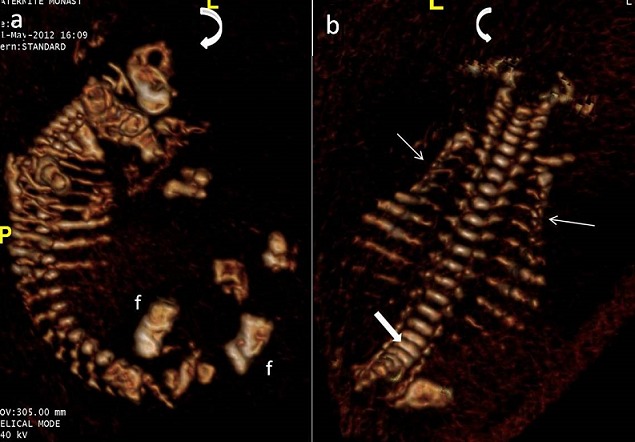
Radiographie du squelette fœtal. f: fémur, t: tibia: défaut d'ossification du crâne, membres très courts et incurvé en receveur téléphonique, côtes déformées porteuses de cals
